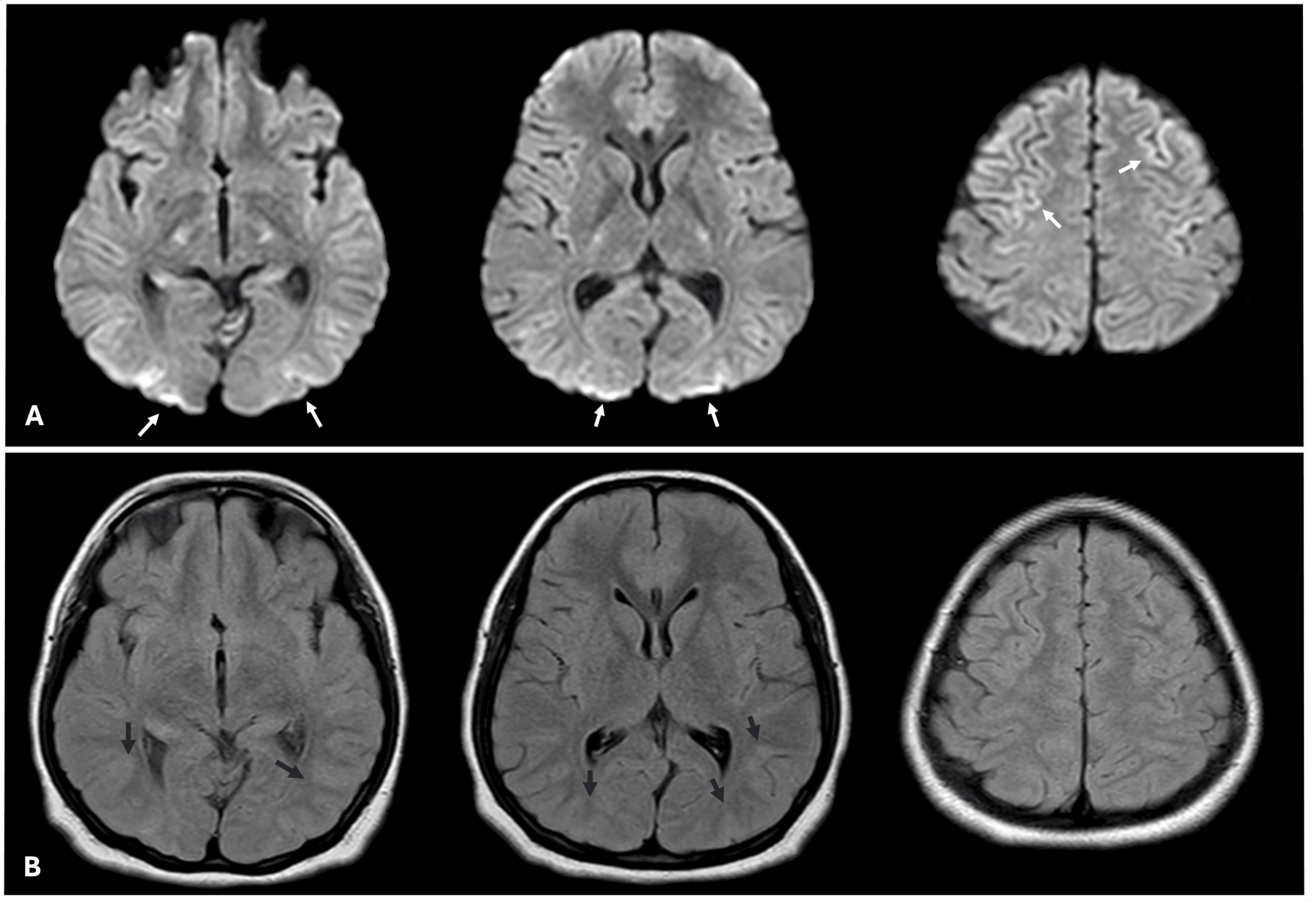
MRI findings on day 1. Faint bilateral occipital and high parietal hyperintensities (white arrows) are seen on axial DWI (A) images. Corresponding FLAIR (B) images show bilateral occipital gyral swelling (black arrows). DWI: diffusion-weighted imaging, FLAIR: fluid-attenuated inversion recovery, MRI: magnetic resonance imaging.
